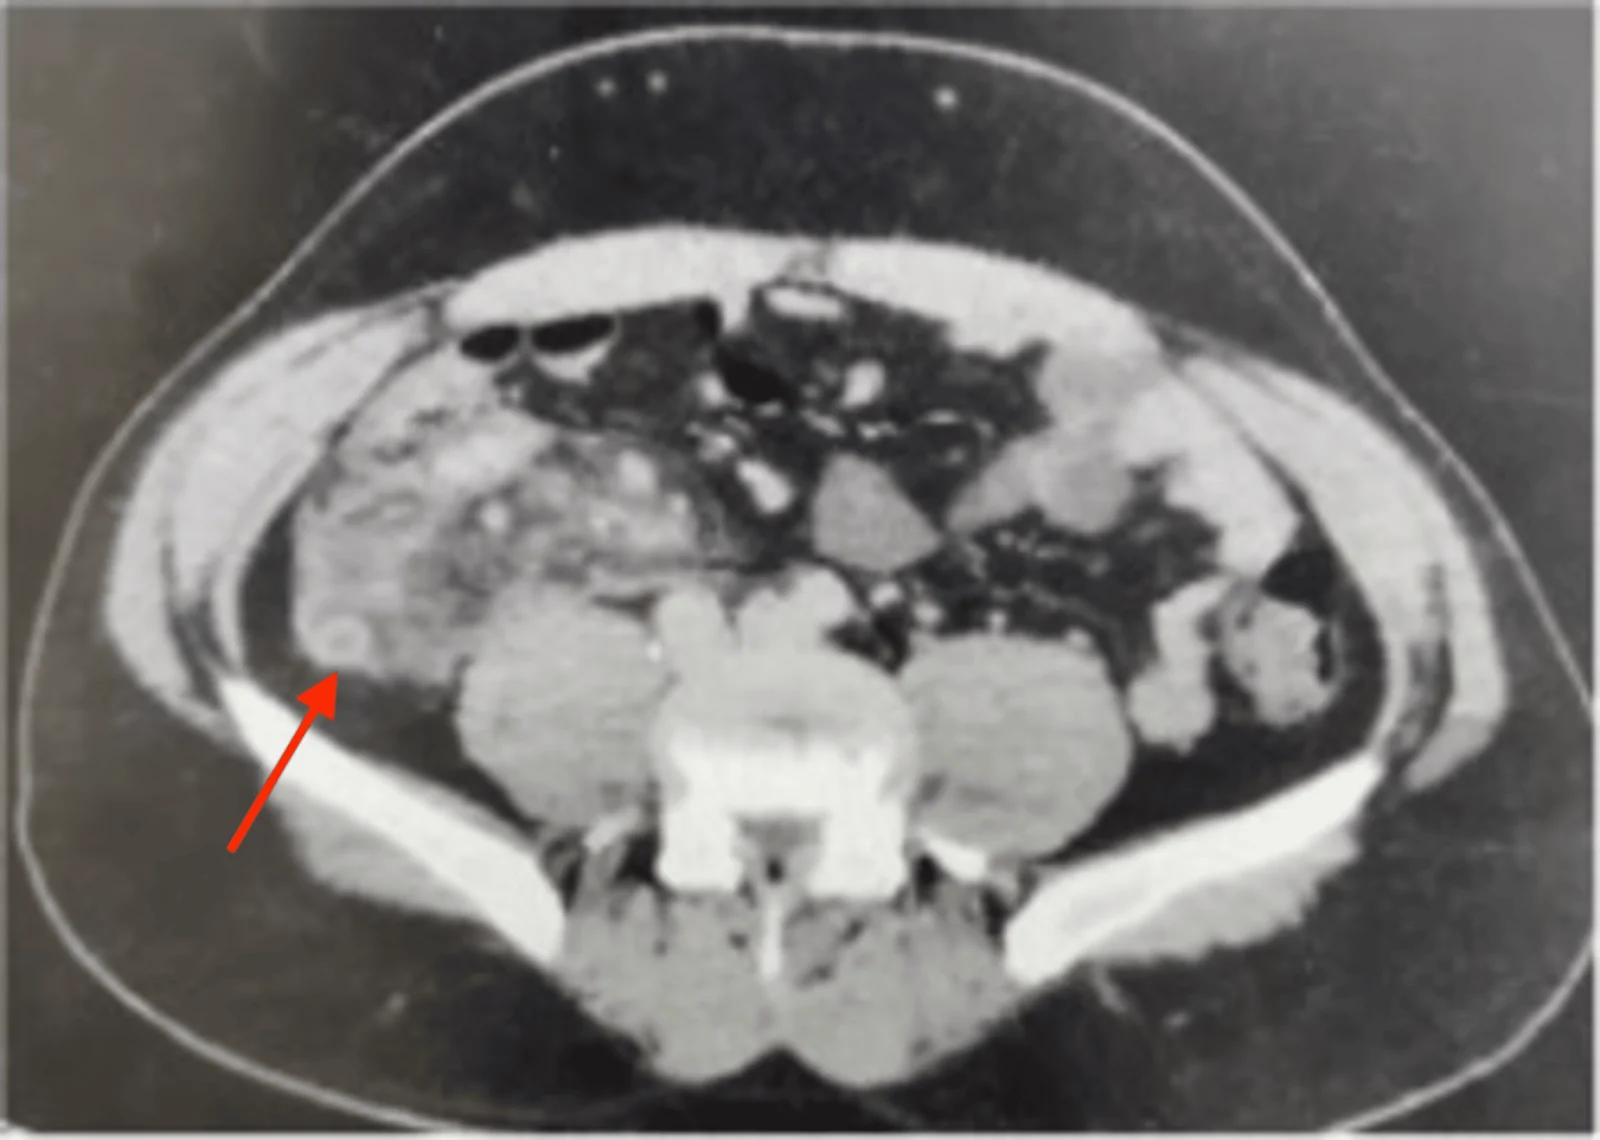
Appendix on CT scan, axial view. The red arrow indicates the inflamed appendix surrounded by significant fat stranding.
